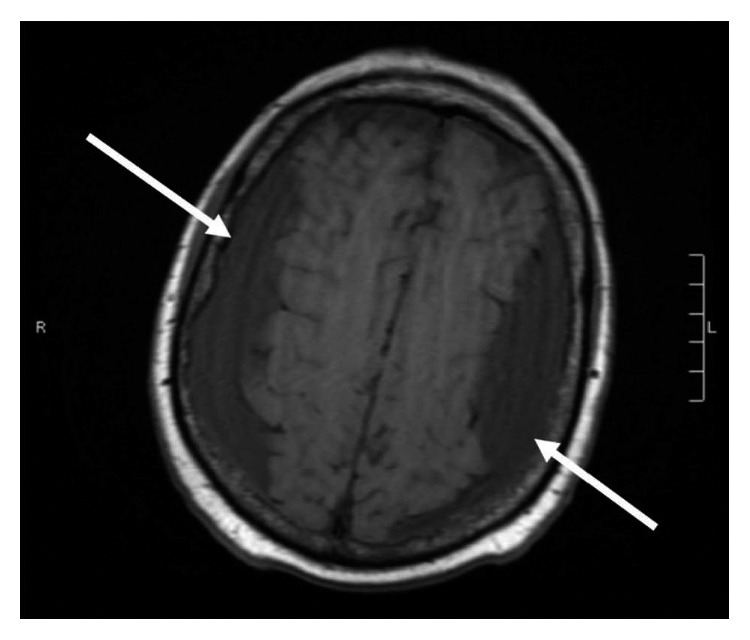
Axial T1 sequence from magnetic resonance imaging (MRI) of the brain.
